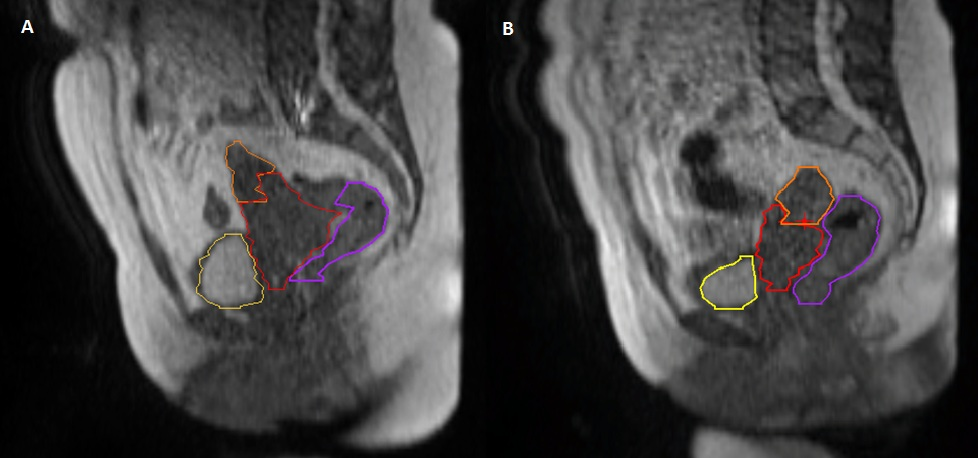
Daily set-up magnetic resonance imaging (MRI) for fraction 1 and 25. Daily set-up imaging using an on-board 0.35-Tesla MRI scanner and a balanced steady state free precession sequence in the sagittal plane for (A) fraction 1 and (B) fraction 25. The bladder (yellow), rectum (purple), gross tumor volume (GTV) (red), and uterus (orange) were contoured on each daily setup MRI. Note the significant reduction in the GTV size, as well as the change in uterine position reflecting inter-fractional motion.
